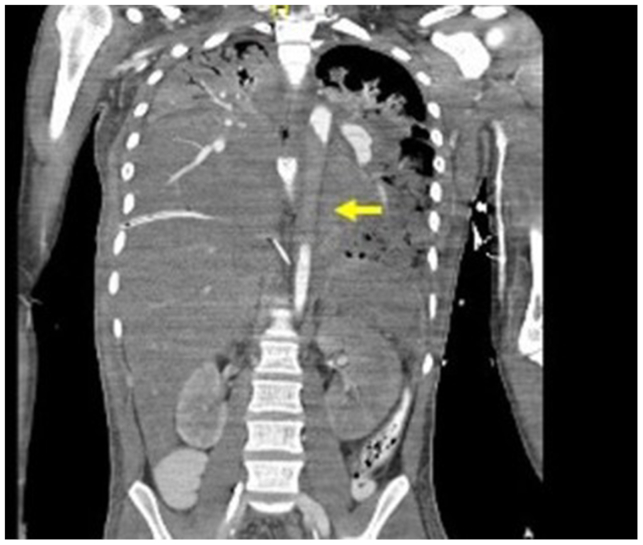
Repeat computed tomography scan demonstrating occlusion of the thoracic aorta.
